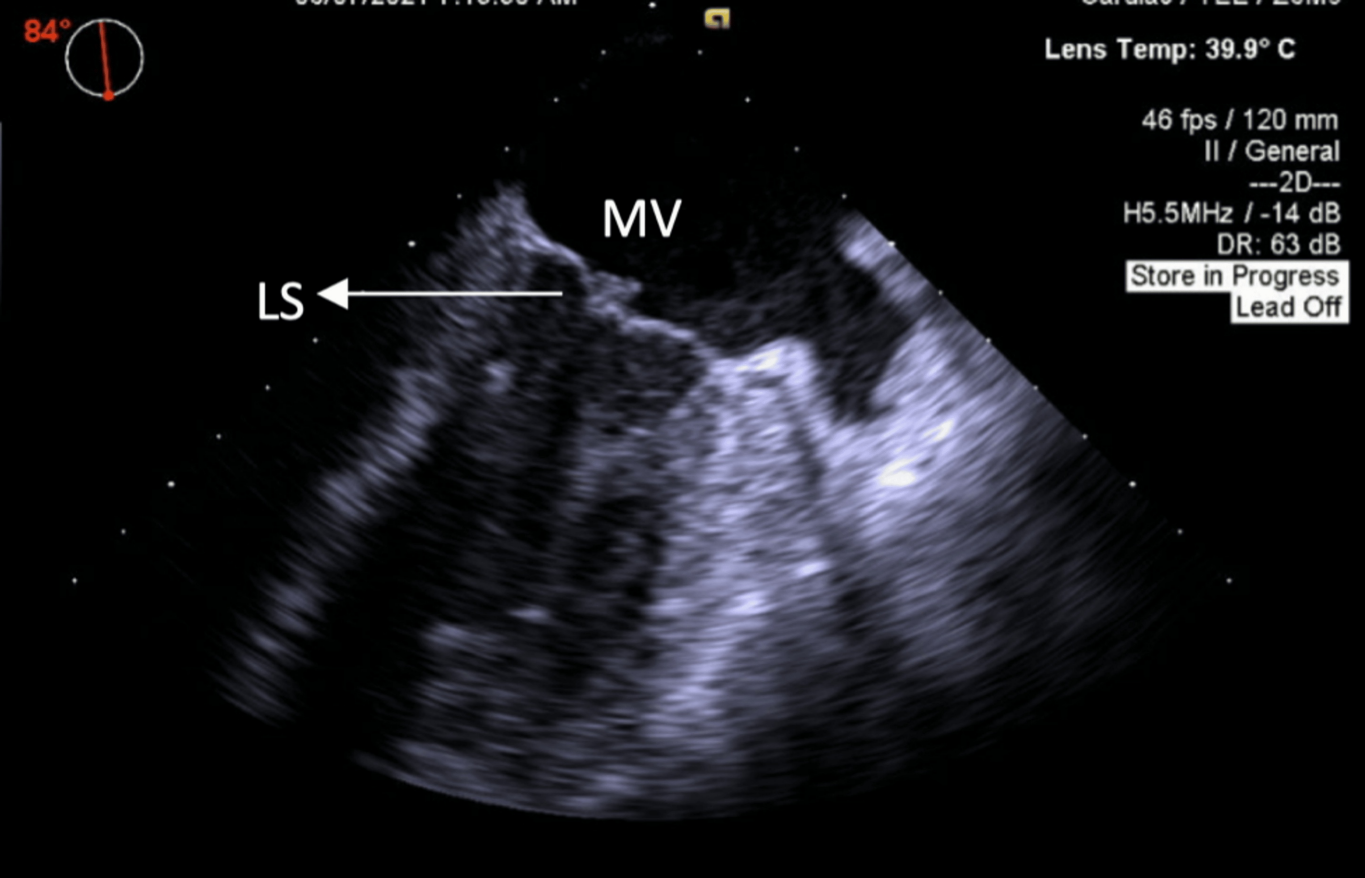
TEE confirming LS on the atrial side of the posterior mitral leaflet LS, Libman-Sacks endocarditis; MV, mitral valve; TEE, transesophageal echocardiogram
